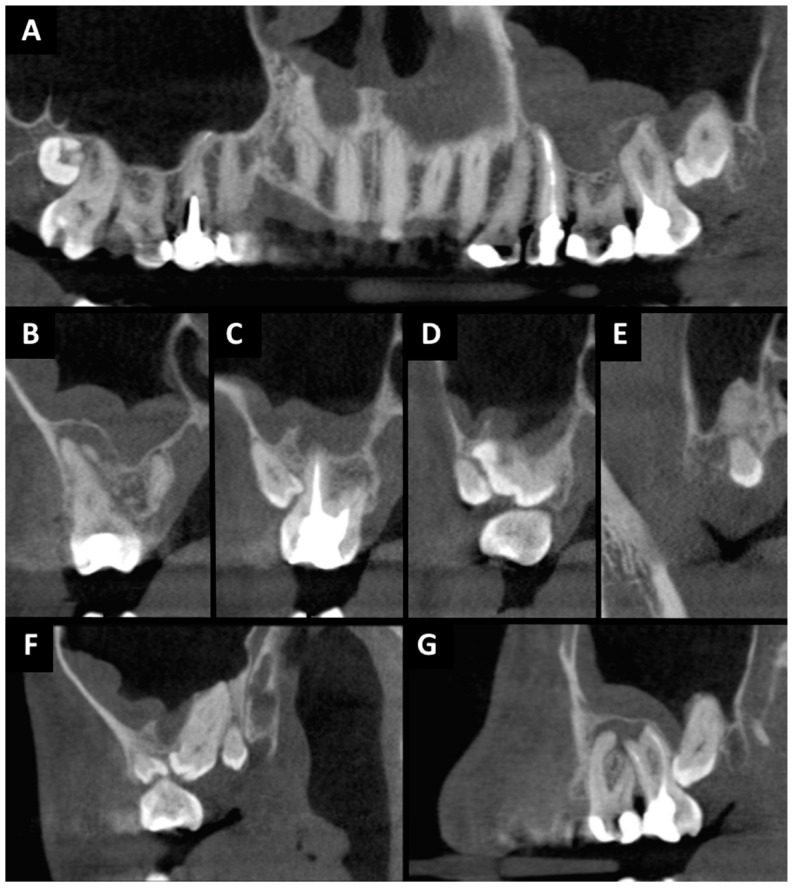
CBCT before surgery treatment: (A) pantomographic reconstruction: chronic odontogenic sinusitis on left side with odontogenic cyst related to tooth 27; (B) cross-sectional view: tooth 27 region; (C) cross-sectional view: tooth 27 and 29′ region; (D) cross-sectional view: tooth 27, 28 and 29′ region; (E) cross-sectional view: tooth 29″ region; (F) sagittal view: tooth 27, 28, 29′ and 29″ region; (G) sagittal view: tooth 26, 27 and 28 region.
